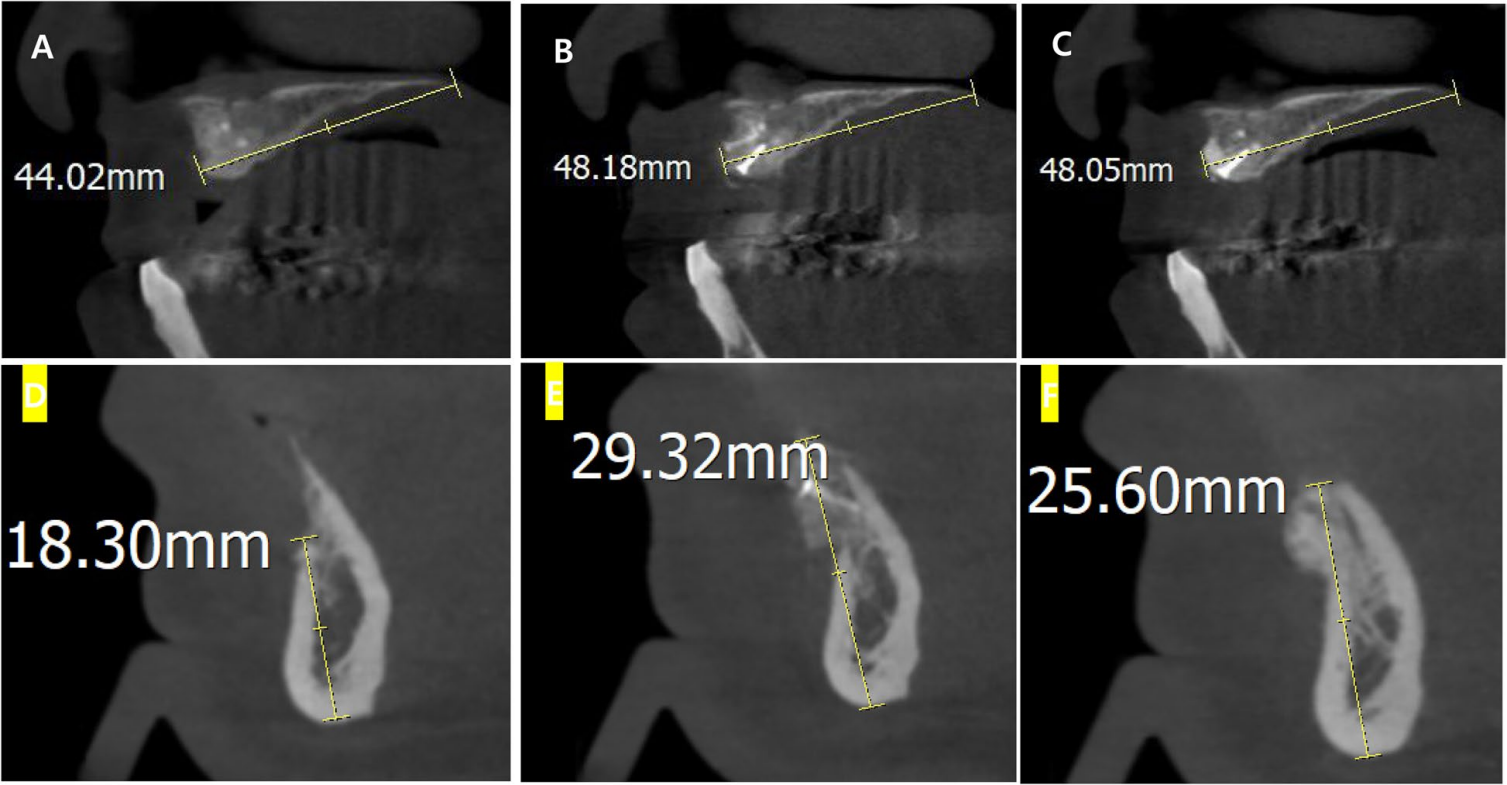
Measuring the amount of increase in bone height or width at the graft site via CBCT. A and D Preoperative CBCT images. B and E Postoperative CBCT images right after surgery. C and F Postoperative CBCT images 6 months after surgery
